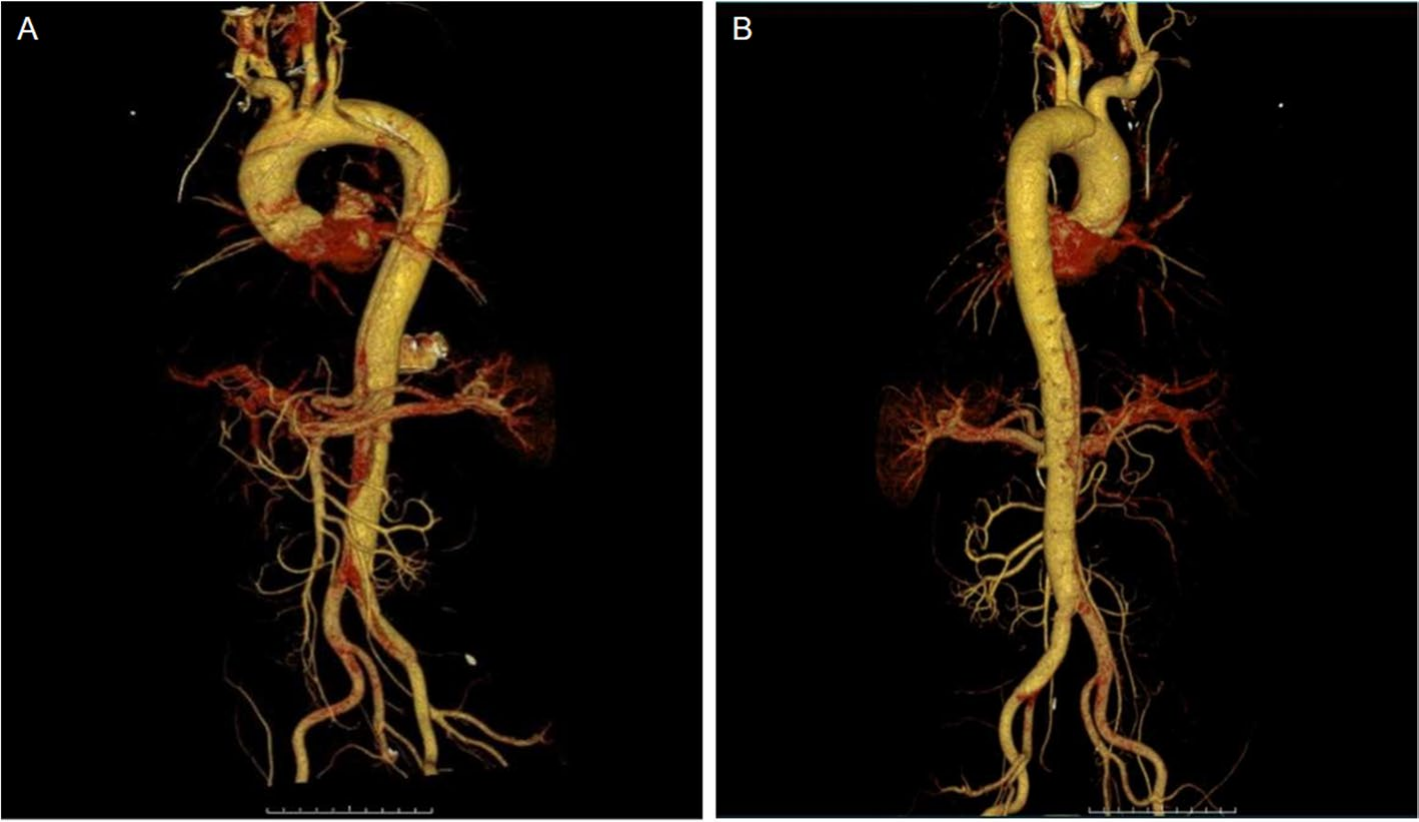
A and B Preoperative computed tomography angiography with 3-dimensional reconstruction of aortic dissection involved the zone 2 and reconstruction of the left common carotid artery was required
